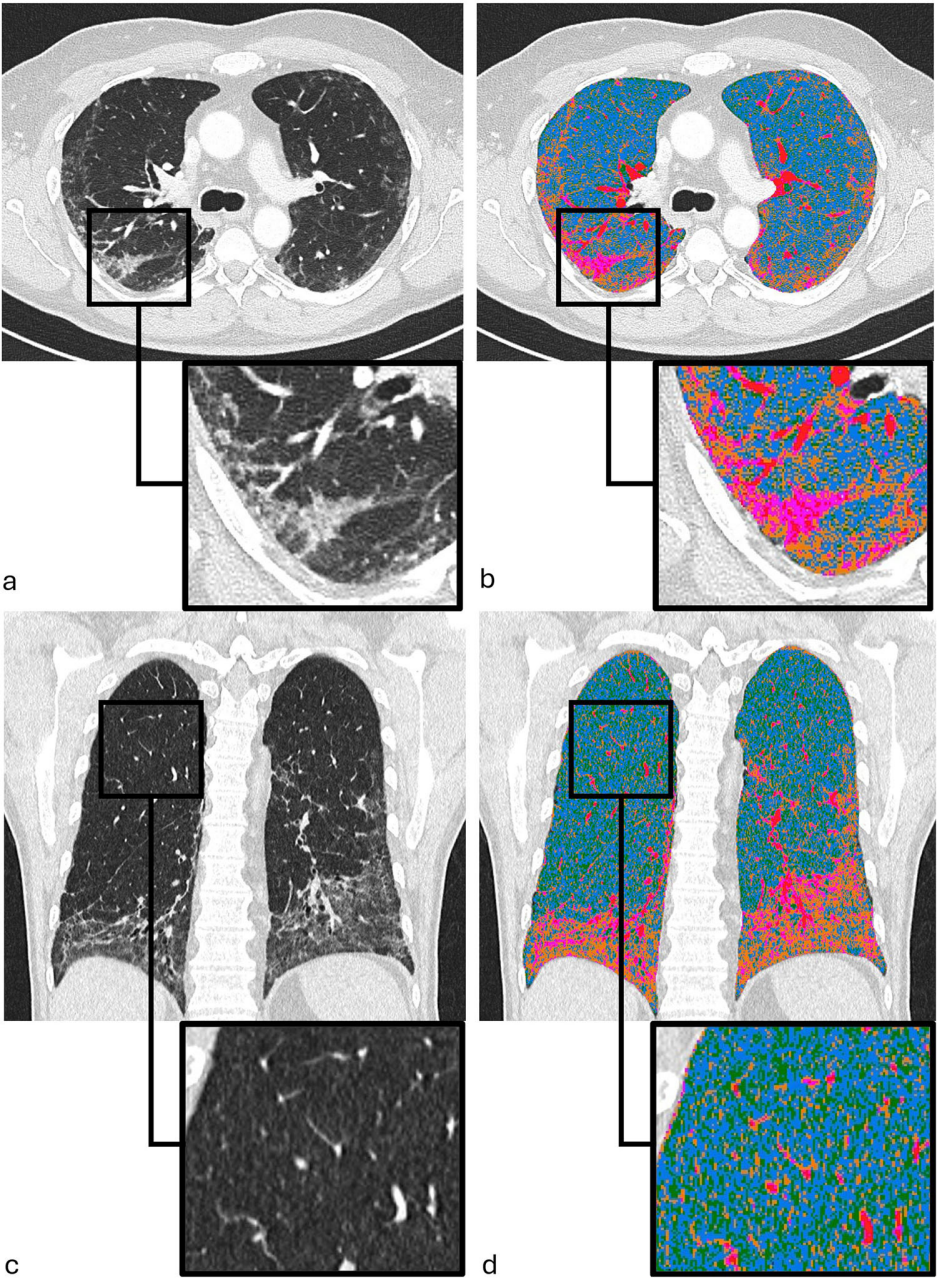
Example of threshold-based quantitative chest CT analysis outputs. a Axial chest CT image demonstrating visually evident consolidation and GGO with an enlarged view of the superior segment of the right lower lobe. b Corresponding output of the quantitative analysis, with an enlarged view of the same region. High-attenuation areas (consolidation) are color-coded in purple, GGO areas in orange, and normal lung parenchyma in blue. Intrapulmonary vessels are shown in red and are excluded from the final quantitative results. c Coronal CT reconstruction showing visually evident centrilobular emphysema, predominantly in the upper lung zones, with an enlarged view of the posterior segment of the right upper lobe. Consolidation and GGO are also seen in the lower lung regions. d Corresponding output of the quantitative analysis, with low-attenuation areas (emphysema) shown in green and normal lung in blue, along with an enlarged view of the posterior right upper lobe segment. CT, computed tomography; GGO, ground-glass opacity
